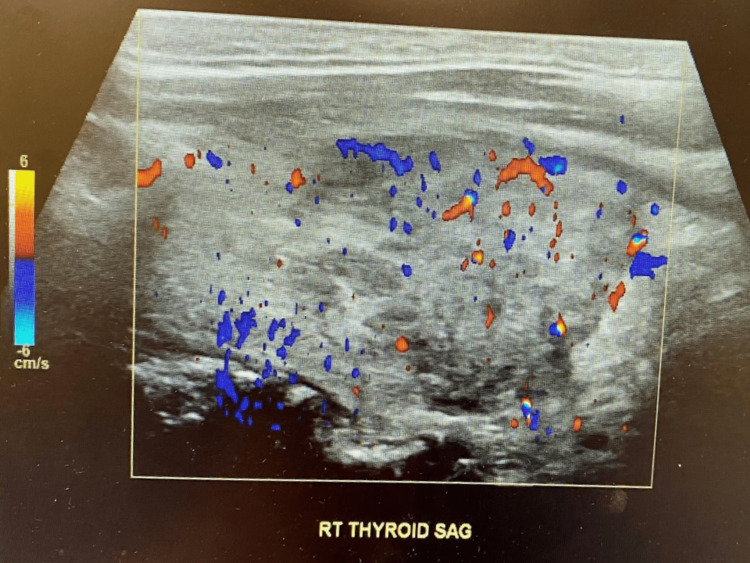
Ultrasound of enlarged heterogeneous thyroid with colored stills.
